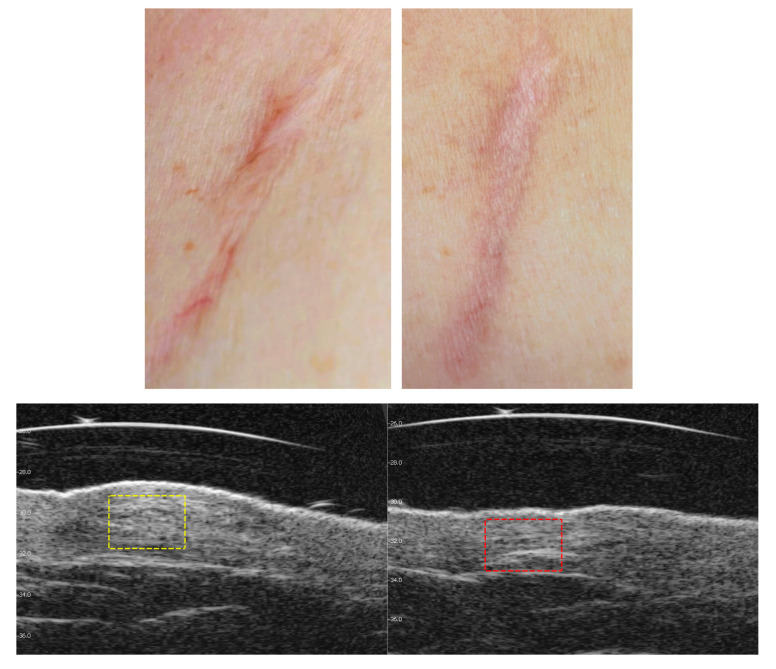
Visible ((upper) photo) and ultrasonographic ((lower) photo) changes before (left) and after (right) scar therapy of patient P2.
